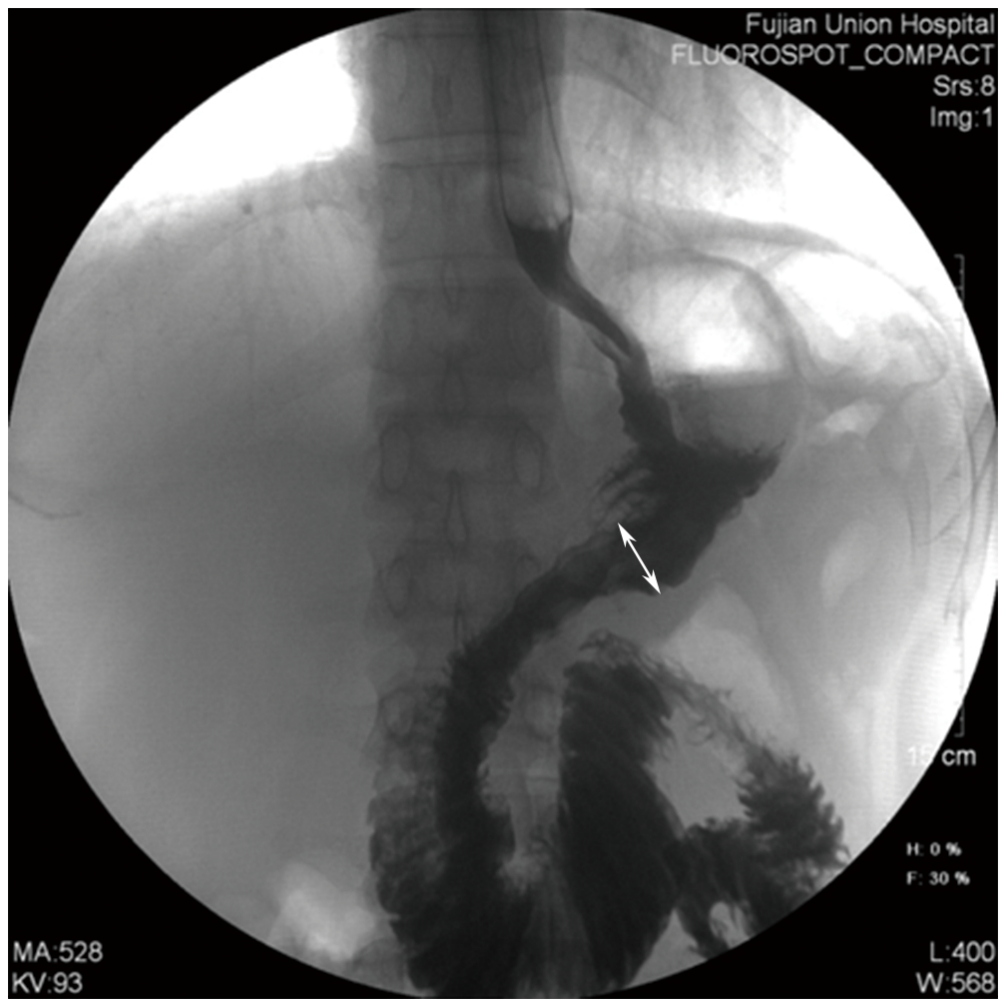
The film of upper gastrointestinal radiography. The film of upper gastrointestinal radiography with diatrizoate meglumine as the contrast medium on postoperative day 7 for one patient underwent the modified delta-shaped gastroduodenostomy. The inner diameter of the anastomosis was measured the length of the white arrow as shown in the figure.
